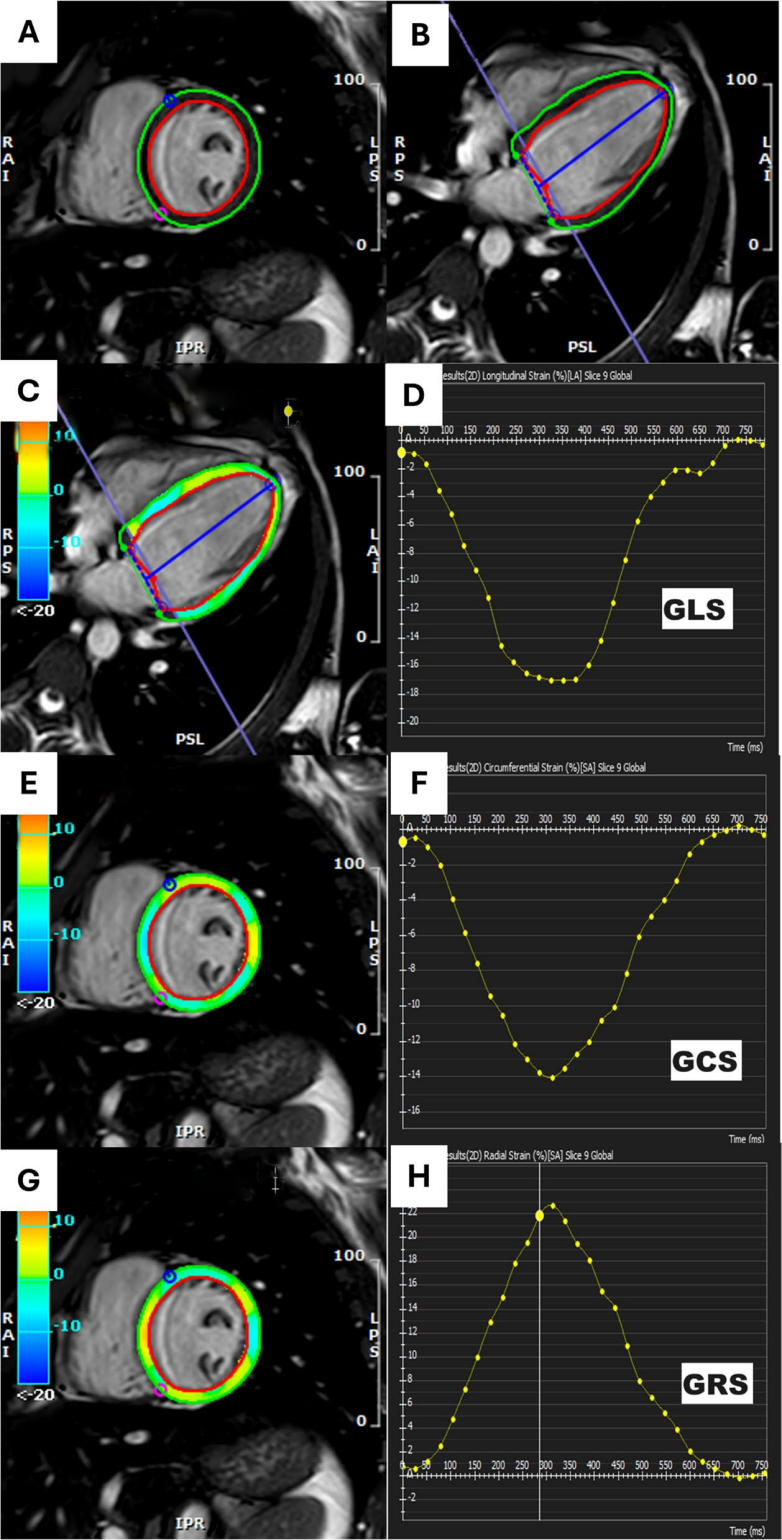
Feature traking analysis in a patient with β-thalassaemia major (A, B) Analysis of cardiac magnetic resonance imaging feature tracking strain of short-axis and four-chamber cine images. Color map and curve of (C, D) global longitudinal strain (GLS), (E, F) global circumferential strain (GCS), and (G, H) global radial strain (GRS) in a patient with thalassaemia major with preserved left ventricular ejection fraction
